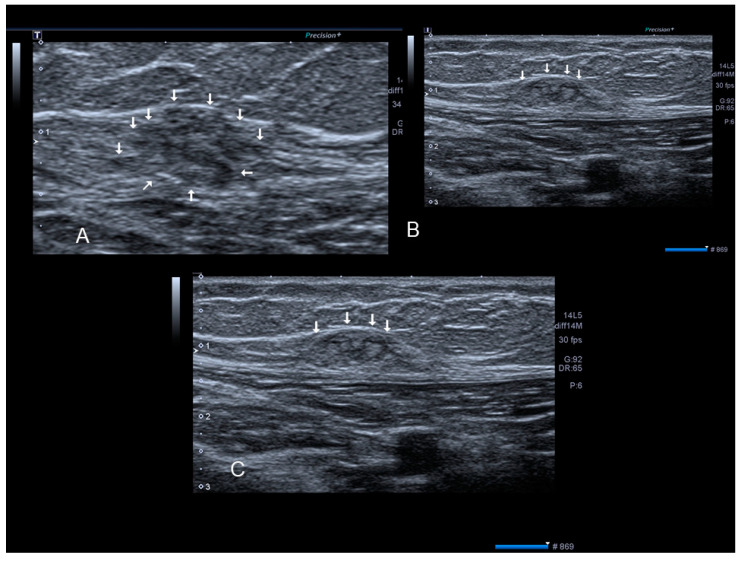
Ultrasonographic images of one nodule (A–C) (see thin with arrows), of scar endometriosis infiltrating the external oblique muscle in a woman with a previous cesarean section some years before cesarean scar endometriosis.
